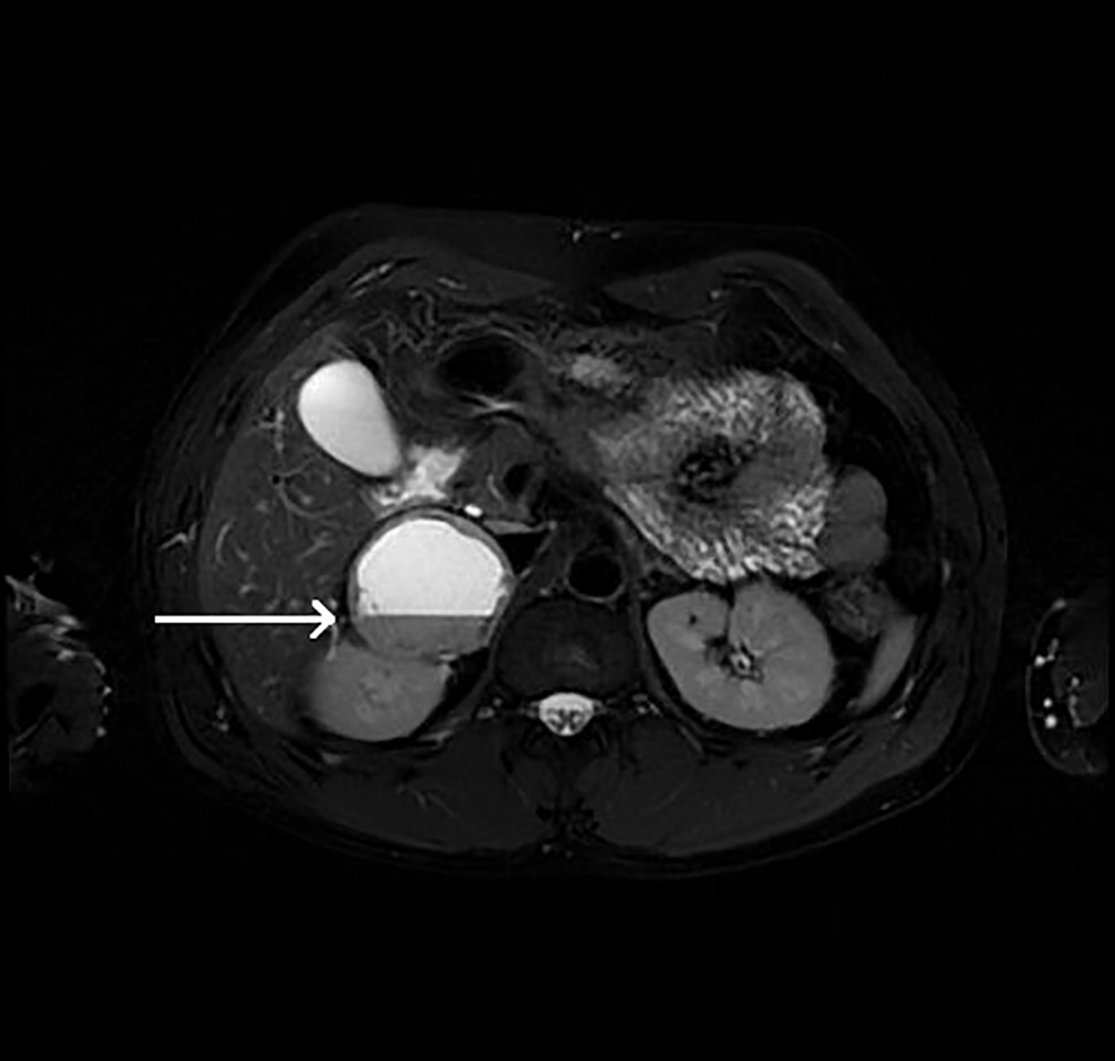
Patient number 5: MRI of the abdomen, T2-weighted axial image – right adrenal lesion with mostly hyperintense signal with fluid-fluid level (thin arrow).
